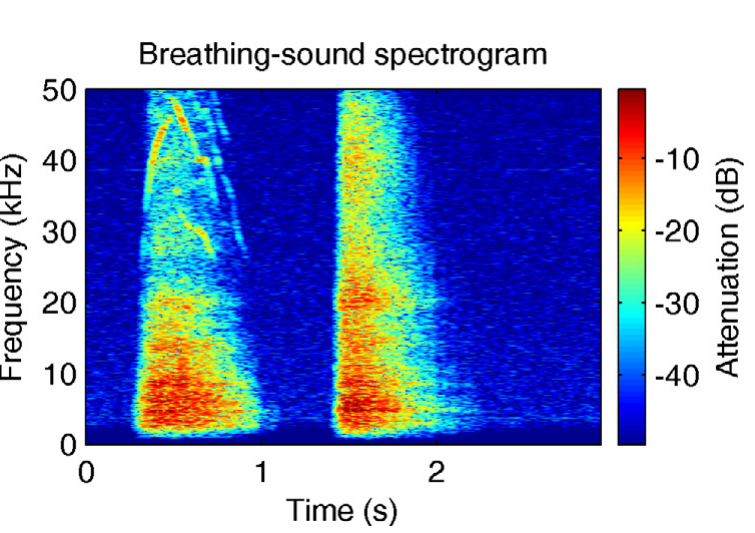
Spectrogram of a recorded breathing sound. The spectrogram shows the spectro-temporal features of an air intake (left) and output (right) in a single breathing-sound cycle. Frequency-modulated, ultrasonic tonal components can be observed during air intake. These components result from turbulences in the nasal cavity during depression. Note the good signal-to-noise ratio, which was realizable with the recording equipment described in the methods.
